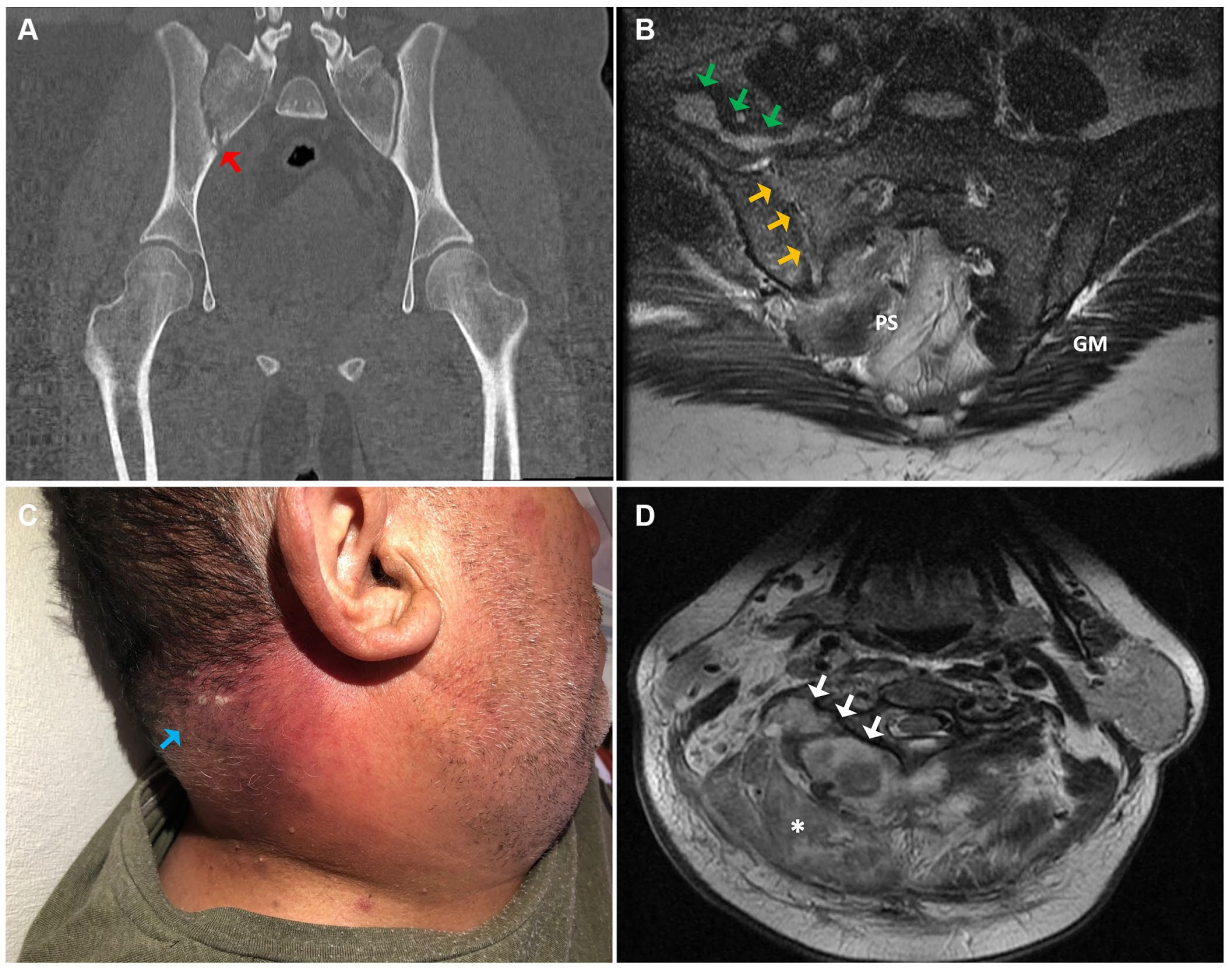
(A) Coronal section of the pelvic computed tomography (CT) scan, showing bone erosions and fragmentation of the right sacroiliac joint (red arrow). (B) Axial section of the pelvic (T2-weighted MRI) evidencing synovitis, osteitis, and erosions of the right sacroiliac joint (yellow arrows) in continuity with periarticular collections (green arrows). (C) An erythematous plaque with multiple pustules in the posterior cervical region concordant with staphylococcal anthrax (light blue arrow). (D) A deep right paravertebral cervical abscess is observed on the axial T2-weighted MRI (white arrows), associated with increased soft tissue signal congruent with a phlegmon (asterisk). PS, presacral space; GM, gluteus maximus.
